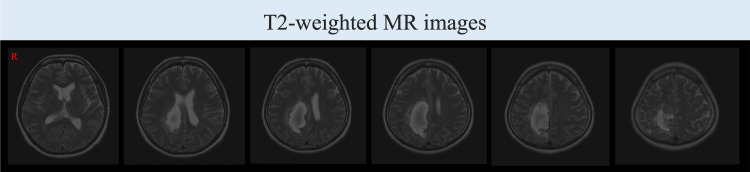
T2-weighted brain MR showed a high-signal response in a wide area centered on the vertex
